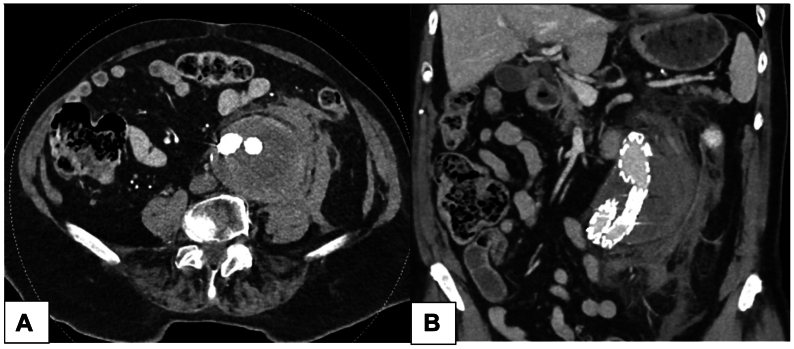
Computed tomography (CT) angiogram showing axial (A) and coronal (B) views of contained abdominal aortic aneurysm (AAA) rupture after endovascular aneurysm repair (EVAR).
